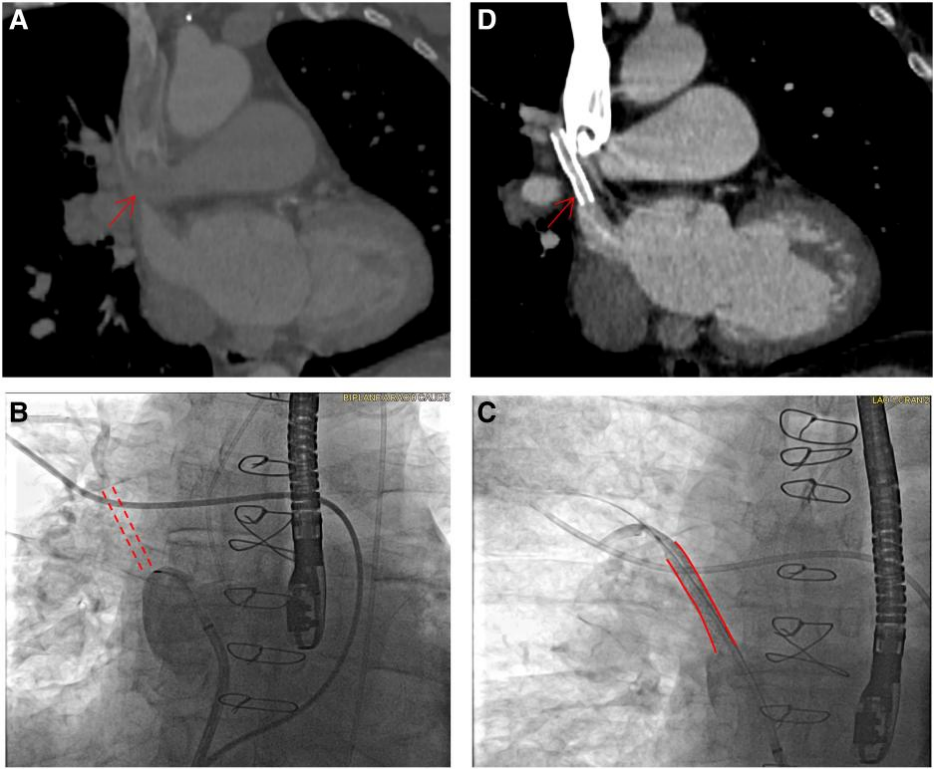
Case 1. (*A*) Computed tomography scan 6 months post-surgery showing obstructed baffle (arrow). (*B*) Fluoroscopy showing obstructed baffle (dotted lines). (*C*) Fluoroscopy showing patent baffle post-stenting (solid lines). (*D*) CT at 39 months post-stenting showing patent baffle.
